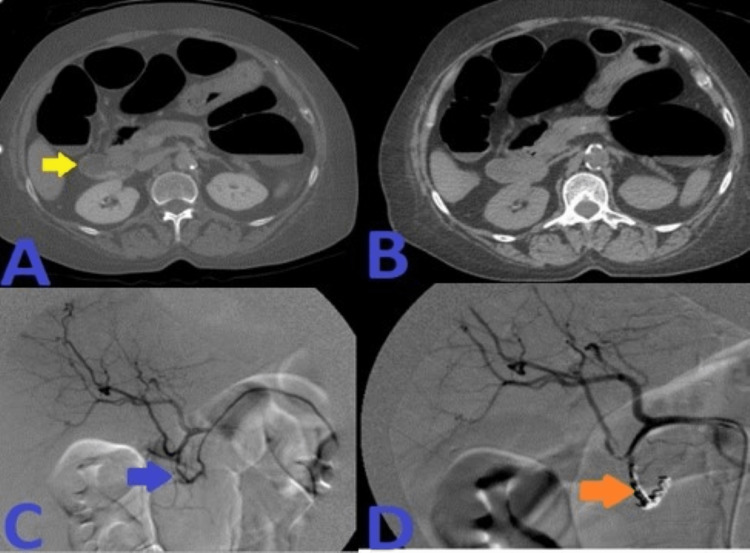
A: CT angiogram abdomen with contrast showed hyperdense material seen on the portal venous phase within the second portion of the duodenum, not present on the noncontrast phase of the CT as seen in image B. C: Super-selective GDA angiograms using microcatheter system demonstrated duodenal culprit bleeding from lateral superior branch of the GDA. D: Post-embolization selective and super-selective angiograms demonstrated no evidence of active bleeding signs, and complete resolution of the above findings with four deployed coils in appropriate positions, just beyond the takeoff of an accessory right hepatic artery coming off as anatomical variant from the GDA. CT: computed tomography; GDA: gastroduodenal artery.
